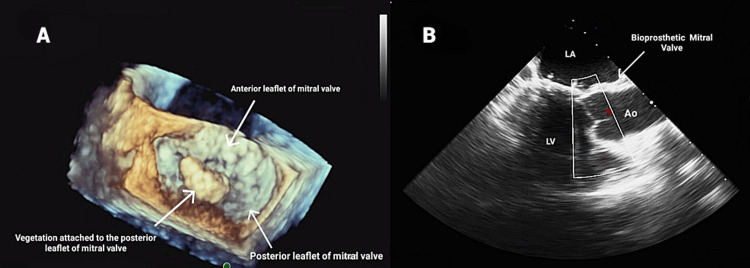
(A) Surgeon’s view of TEE showing vegetation attached to the posterior leaflet of the mitral valve. (B) TEE long axis showing the bioprosthetic mitral valve after replacement Ao, aorta; LA, left atrium; LV, left ventricle; TEE, transesophageal echocardiography
